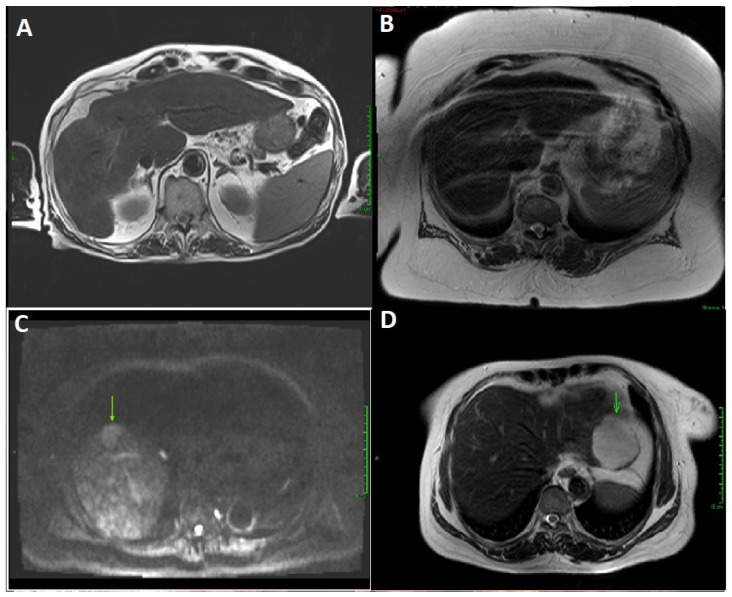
Abbreviated MRI images from study participants. (A). Satisfactory quality abbreviated MRI in a patient with ArLD cirrhosis, with no lesion detected. (B). Insufficient quality abbreviated MRI due to breathing artefact in patient with body mass index > 55. (C). Hepatocellular carcinoma (arrow) in a patient with cirrhosis, with no lesion detected on surveillance ultrasound. (D). Haemangioma (arrow) in a patient, with no lesion detected on ultrasound.
